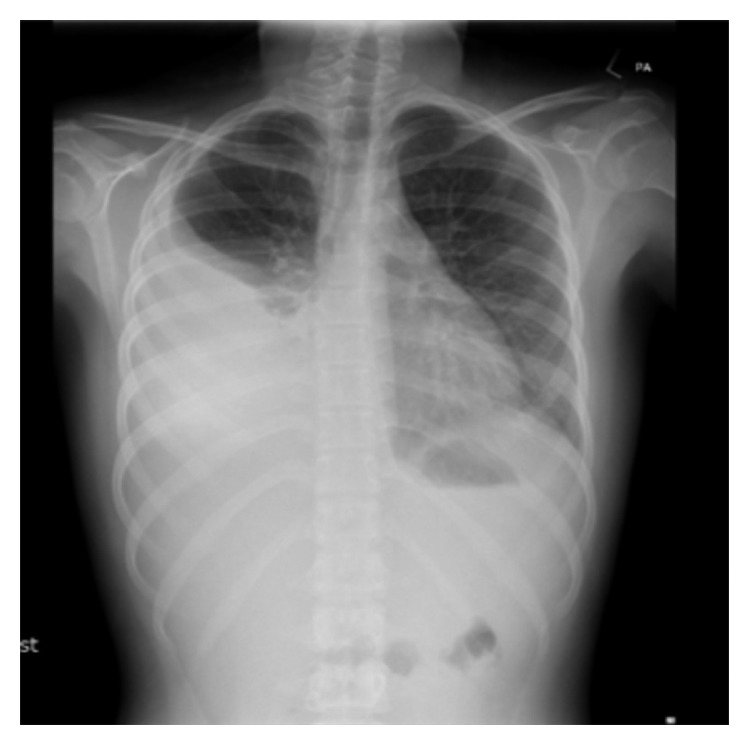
PA erect chest radiograph: large volume right-sided pleural effusion with atelectasis of right mid and lower lobes.
